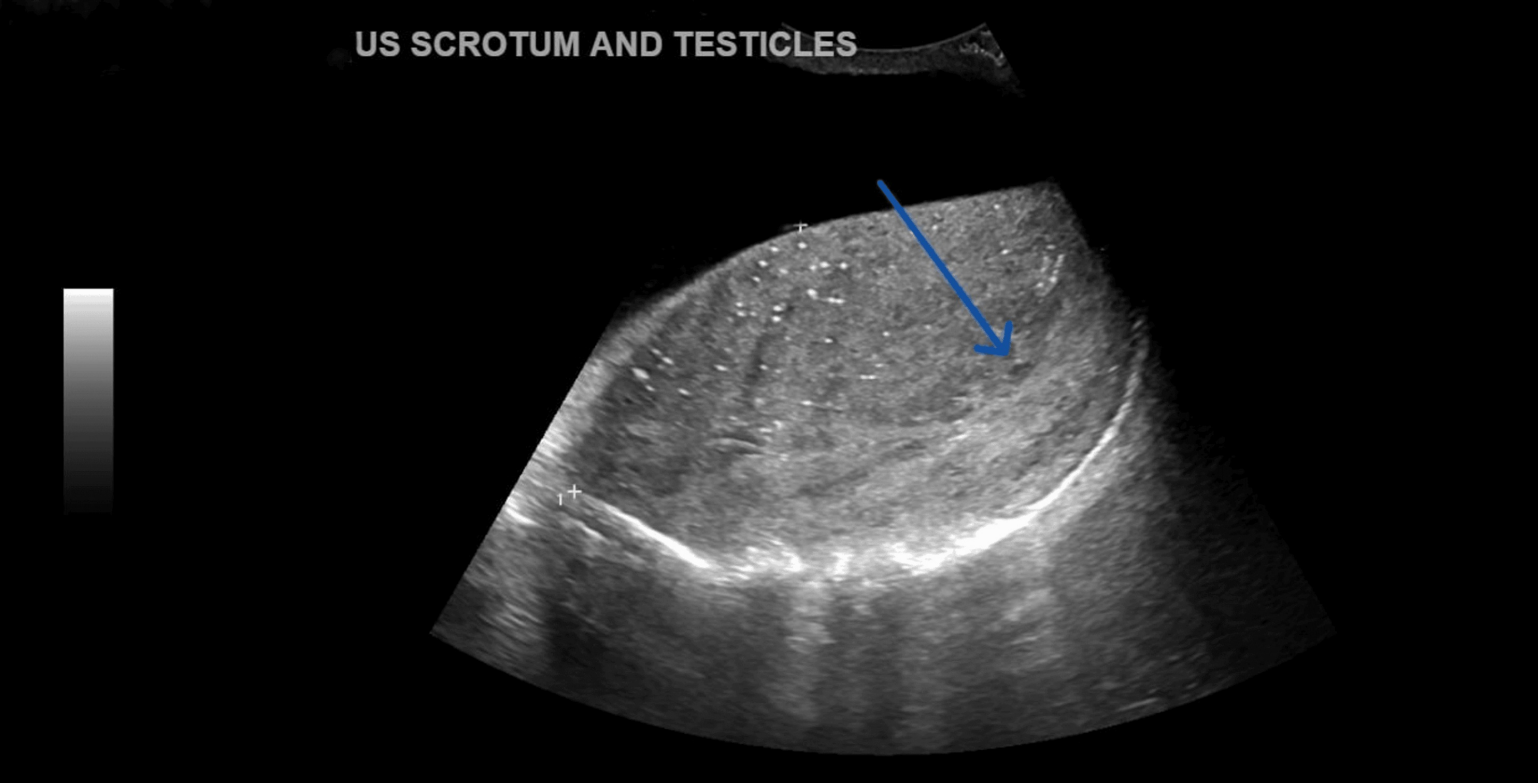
Testicular ultrasound showing left-sided testicular mass (blue arrow) US: ultrasound
